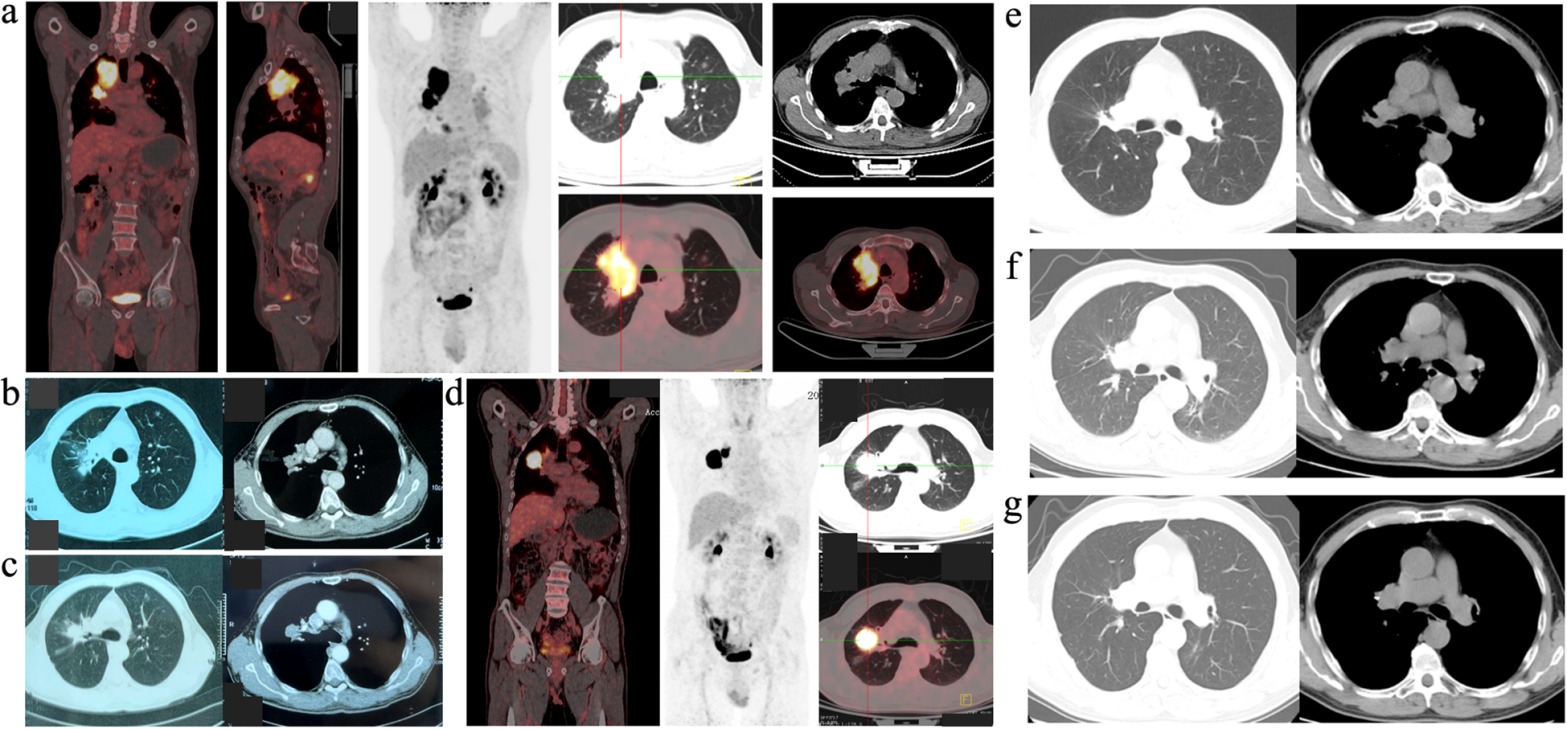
(a) PET-CT of the patient at diagnosis. (b) Enhanced CT after the patients had two cycles of treatment. (c) Enhanced CT after the patients had four cycles of treatment. (d) PET-CT after he had six cycles of treatment. The metabolically active mass was still present, and the SUVmax was about 39.1 and the maximum diameter was 3.5cm plus 3.9cm. (e) Enhanced CT of the hilar lesions after the patient had eight cycles of chemotherapy. (f) Enhanced CT of the hilar lesions after the patient had twelve cycles of chemotherapy. (g) Enhanced CT of the patient in the last follow-up time (March 3, 2020).
